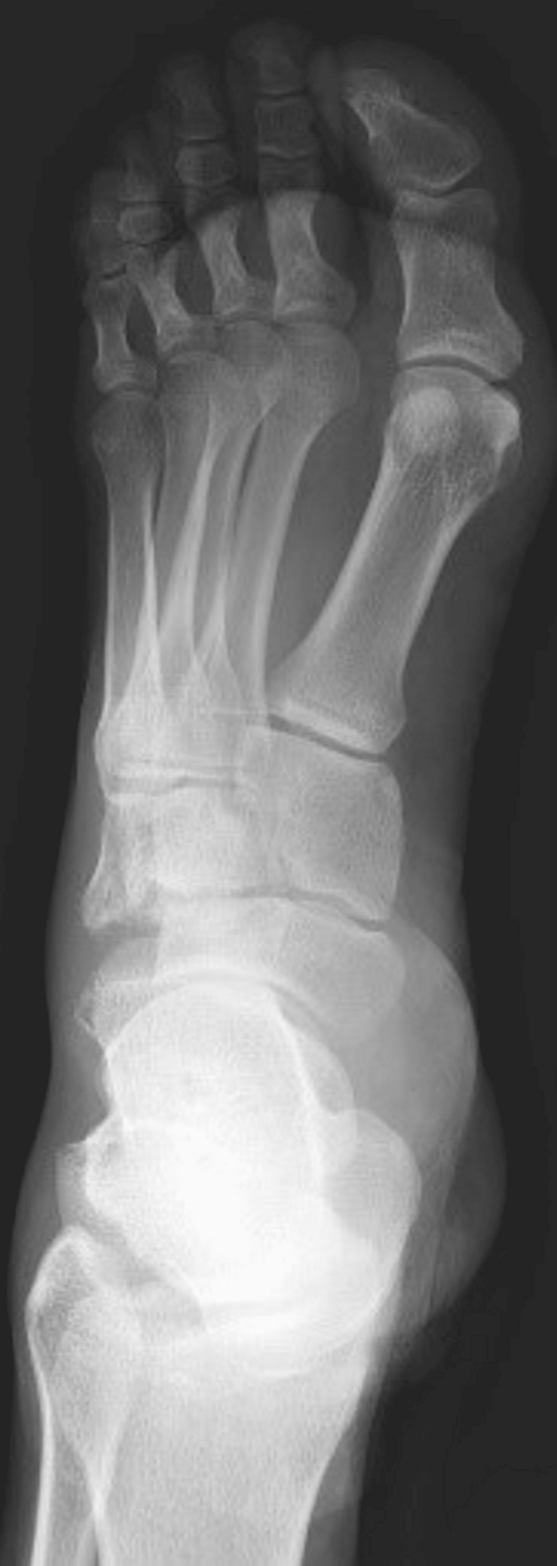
Anteroposterior foot radiograph showing a cuboid fracture
